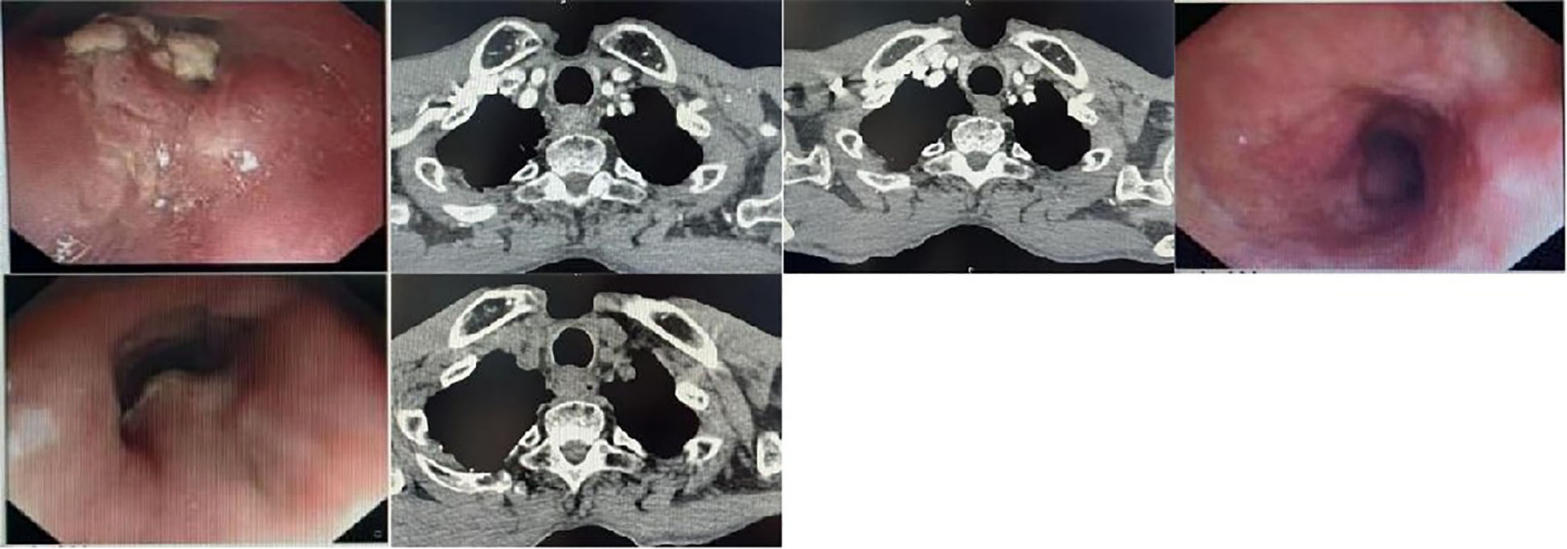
Comparative gastroscopic images and CT at pre-treatment, post-treatment, and recurrence timepoints.
